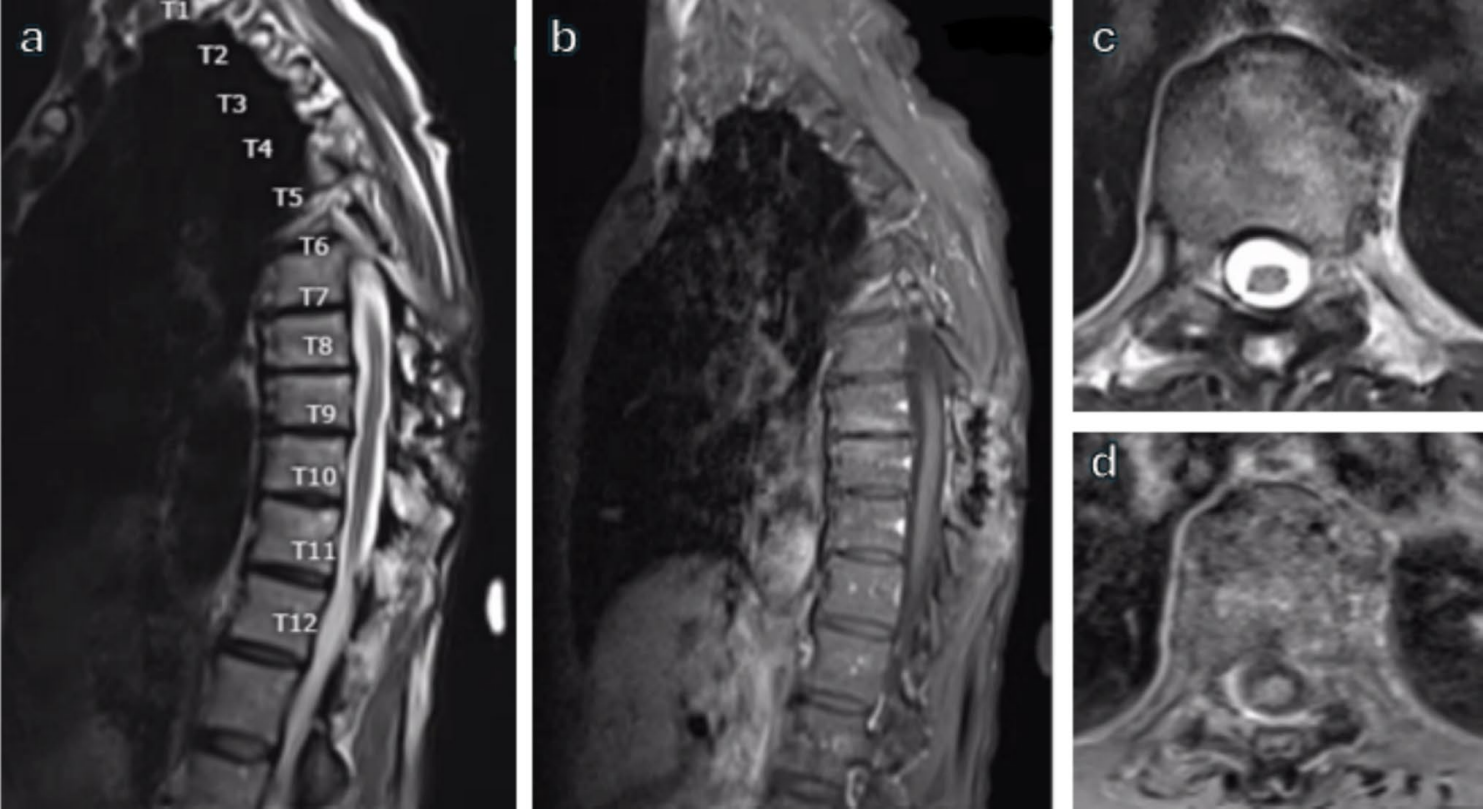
a Sagittal T2, b sagittal T1 post-contrast, c axial T2, and d axial T1 post-contrast MRI of the thoracic spine at 6 month postoperative visit demonstrating no residual or recurrent tumor
